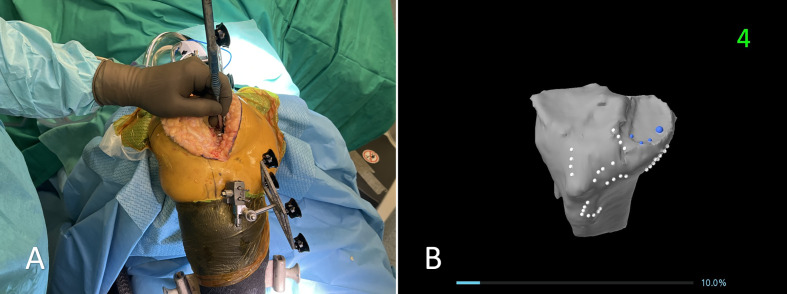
The registration is performed on the tibial component. A: Intraoperative image showing the registration process using a probe to acquire anatomical landmarks on the tibia. The original polyethylene insert has been removed prior to registration, as the preoperative CT scan does not capture it. B: The corresponding visualization on the robotic system’s screen, displaying the registered points on the 3D model of the tibia.
